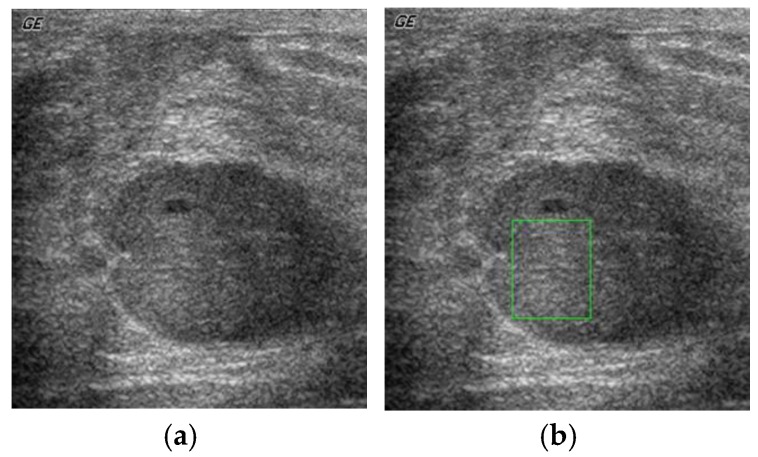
(a) General representation of the ultrasound breast cyst imagery, (b) marked and segregated ROI (region of interest) portions from this imagery.
